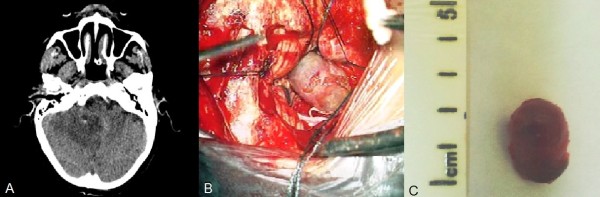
Intra- and post-operative images of the aneurysm. (A) Post-operative computed tomographic scan. (B) Intra-operative photograph of the aneurysm. (C) Photograph of the aneurysm after excision.
